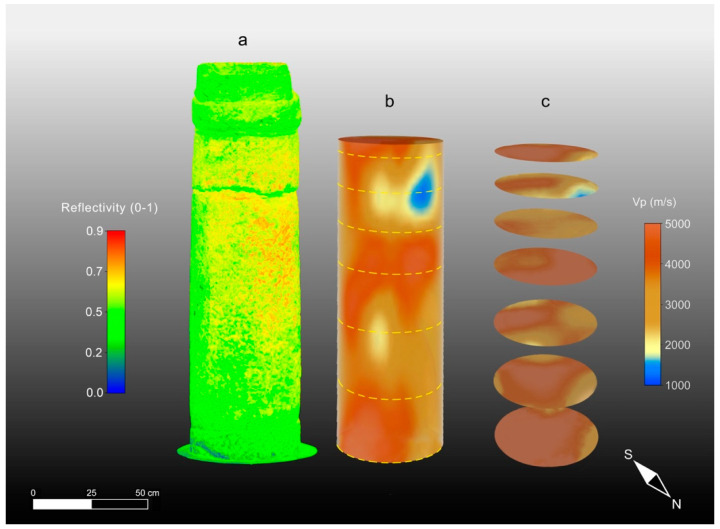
High-resolution 3D models of column 3. (a) TLS reflectivity model; (b) ultrasonic tomography; (c) tomographic slices.
